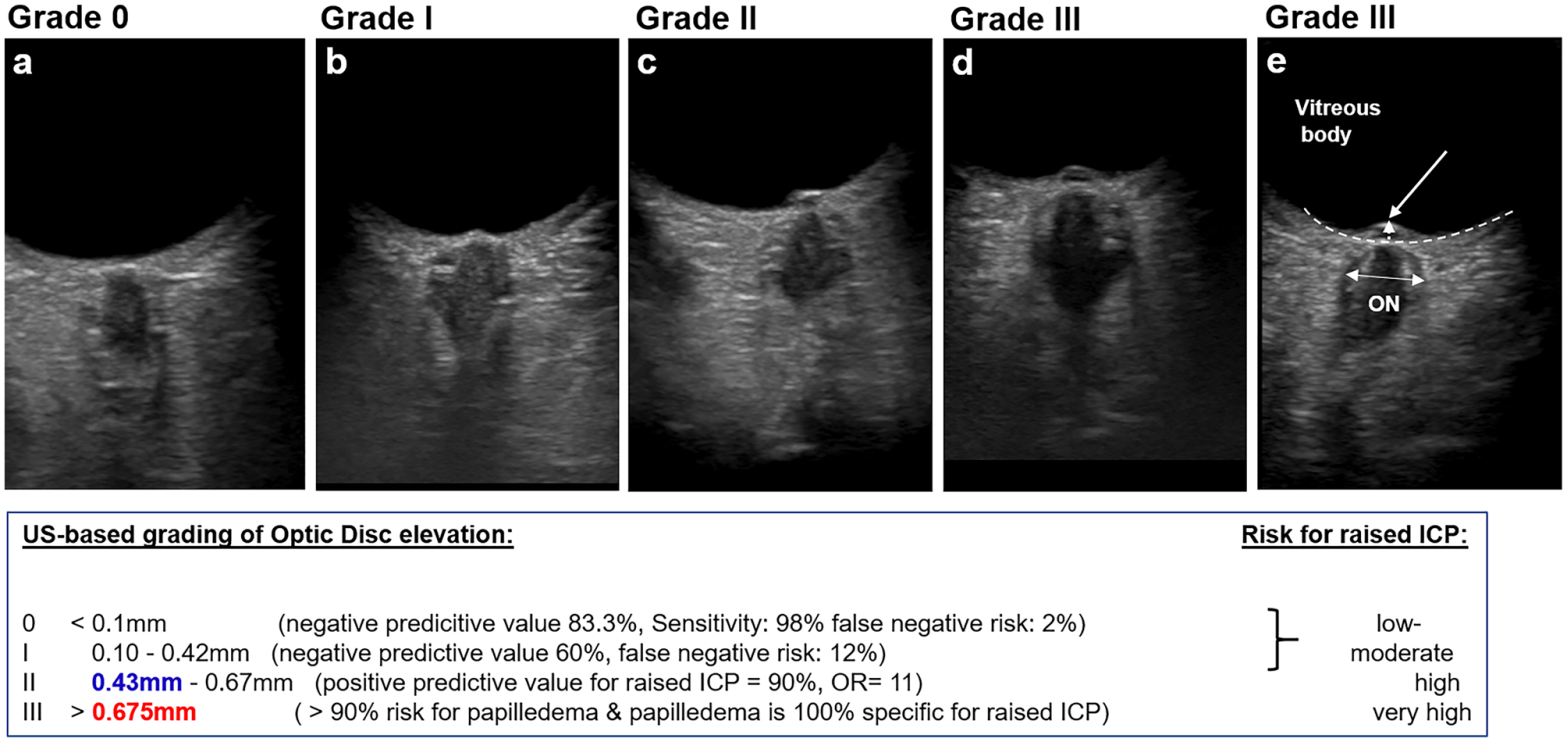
Ultrasound-based grading of optic disc elevation (ODE). a Grade 0 (mean US-ODE 0.087 mm) in patient without papilledema and normal ONSD, no pathology. b Grade I (mean US-ODE 0.23 mm), no papilledema, small ONSD value, no pathology. c Grade II (US-ODE 0.52 mm), no papilledema, but pathology requiring treatment and ONSD enlargement. d, e Grade III (US-ODE 1.21 mm), fundoscopic papilledema, large US-ONSD and diagnosis with need for treatment. e US-based ONSD and ODE measurement. Dotted line marks retina. White double-headed arrow marks area of ONSD measurement. Optic disc elevation is measured from retina at the entrance of the optic nerve (ON) to the maximum of elevation (white arrows) within the vitreous body. a–e show transorbital, B-scan-ultrasonographic images
